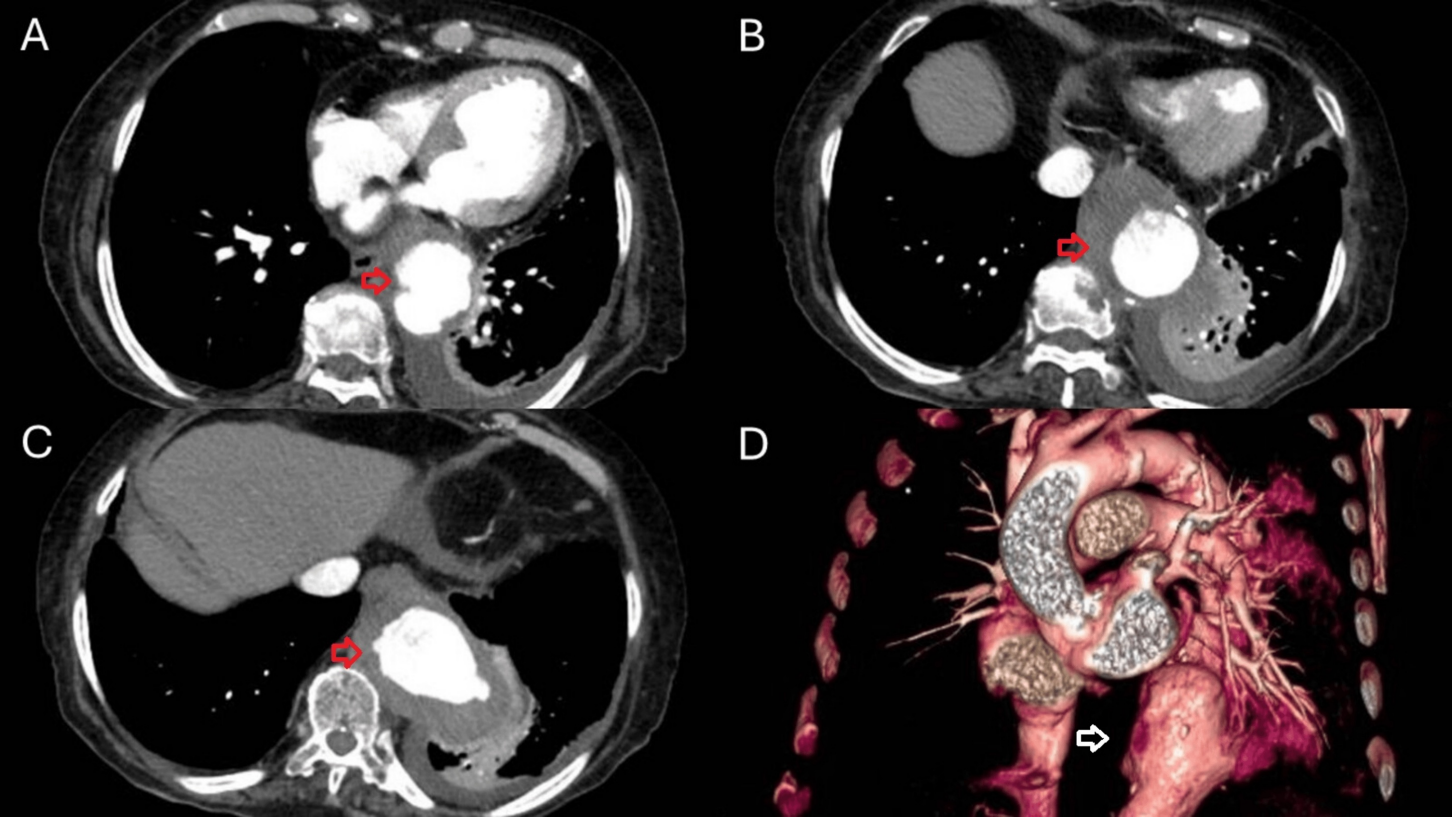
A-C: Contrast-enhanced CT showing aneurysm of the descending and diaphragmatic aorta with a rupture contained by a hematoma adjacent to the medial segment of the left lower pulmonary lobe (red arrows); D: Three-dimensional reconstruction showing aortic aneurysm of the descending aorta (white arrow)
